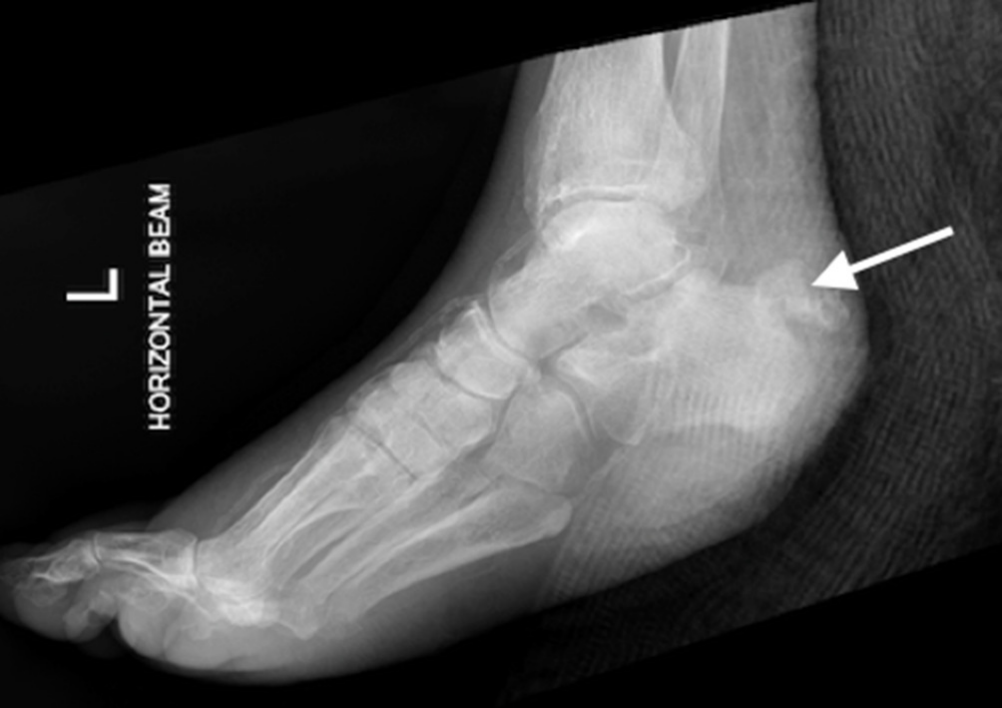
Radiograph of the left foot demonstrates a dressing overlying a heel ulcer and a wedge-shaped calcaneal avulsion fracture (arrow) at the posterior calcaneum.
